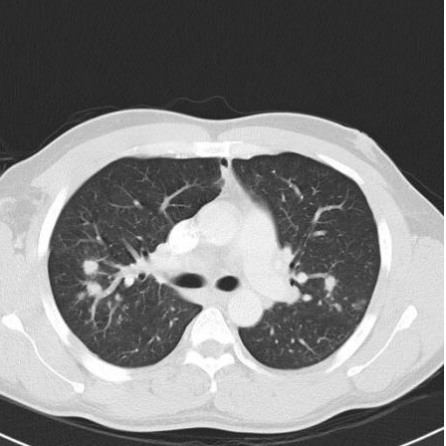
Computed Tomography Scan of the chest showed mediastinal lymphadenopathy and multiple bilateral pulmonary nodules.
